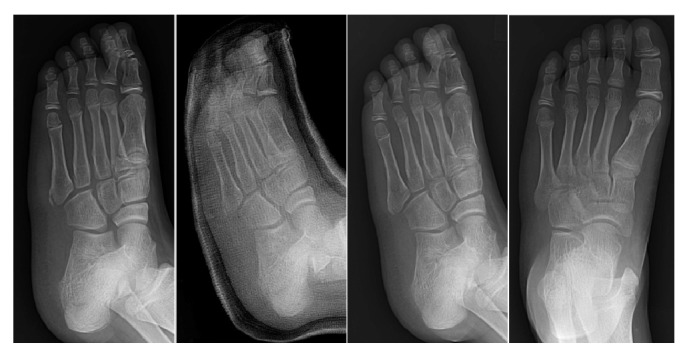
Nonoperative treated, early weight-bearing patient's radiograph. 12-year-old male; radiograph of pre-cast application and 1-, 3-, and 6-month follow-up (from left to right).
